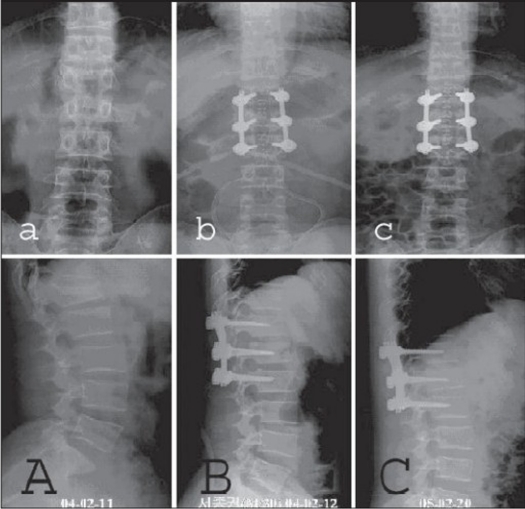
L_1_ burst fracture with retropulsed fragment: (aA) Preoperative plain roentgenograms show collapsed shattered upper 1/2 of L_1_ body with a retropulsed bony fragment and widened pedicle distance. (bB) Immediate postop roentgenograms show restored L_1_ vertebral height and sagittal alignment. (cC) 14 months followup shows fracture consolidated and restored basis height
